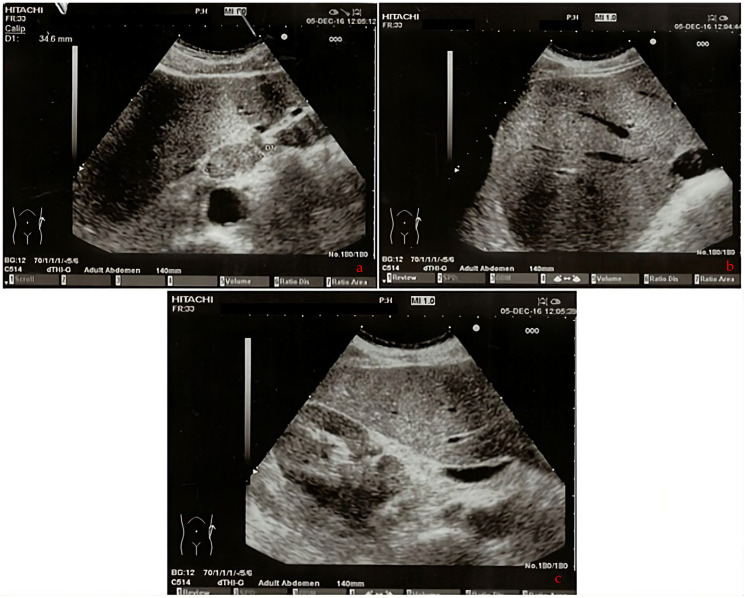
(a–c) The abdomen ultrasound images of perihepatic lymphadenopathy (2016).
